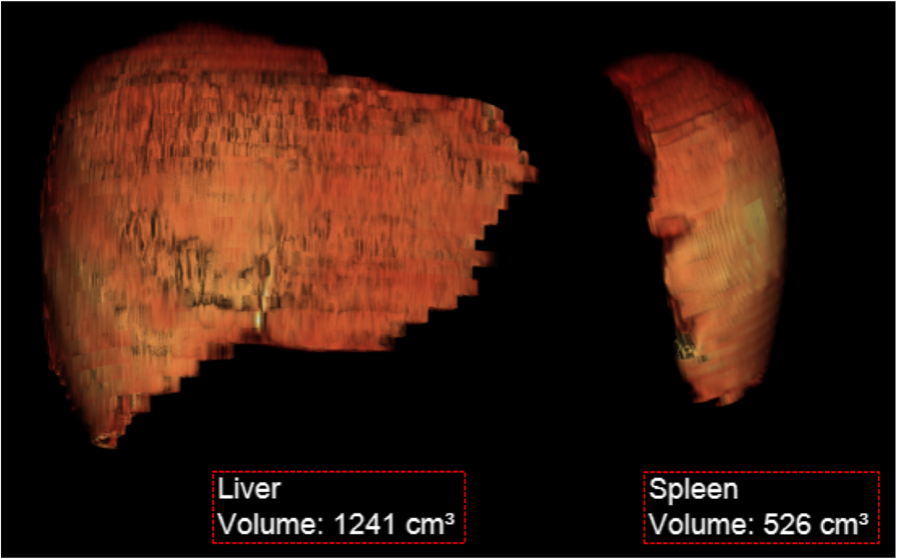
Three-dimensional reconstructions of liver and spleen volumes from MRI in Gaucher disease. Volume-rendered organ volume reconstructions generated from non-contrast MRI data were used to track treatment response in a 9-year-old male with Gaucher disease. Organ volumes for the liver (1241 mL, 1.03 MN) and spleen (526 mL, 5.44 MN) are decreased from a prior examination at 6 years of age (1.35 MN and 6.43 MN, respectively)
